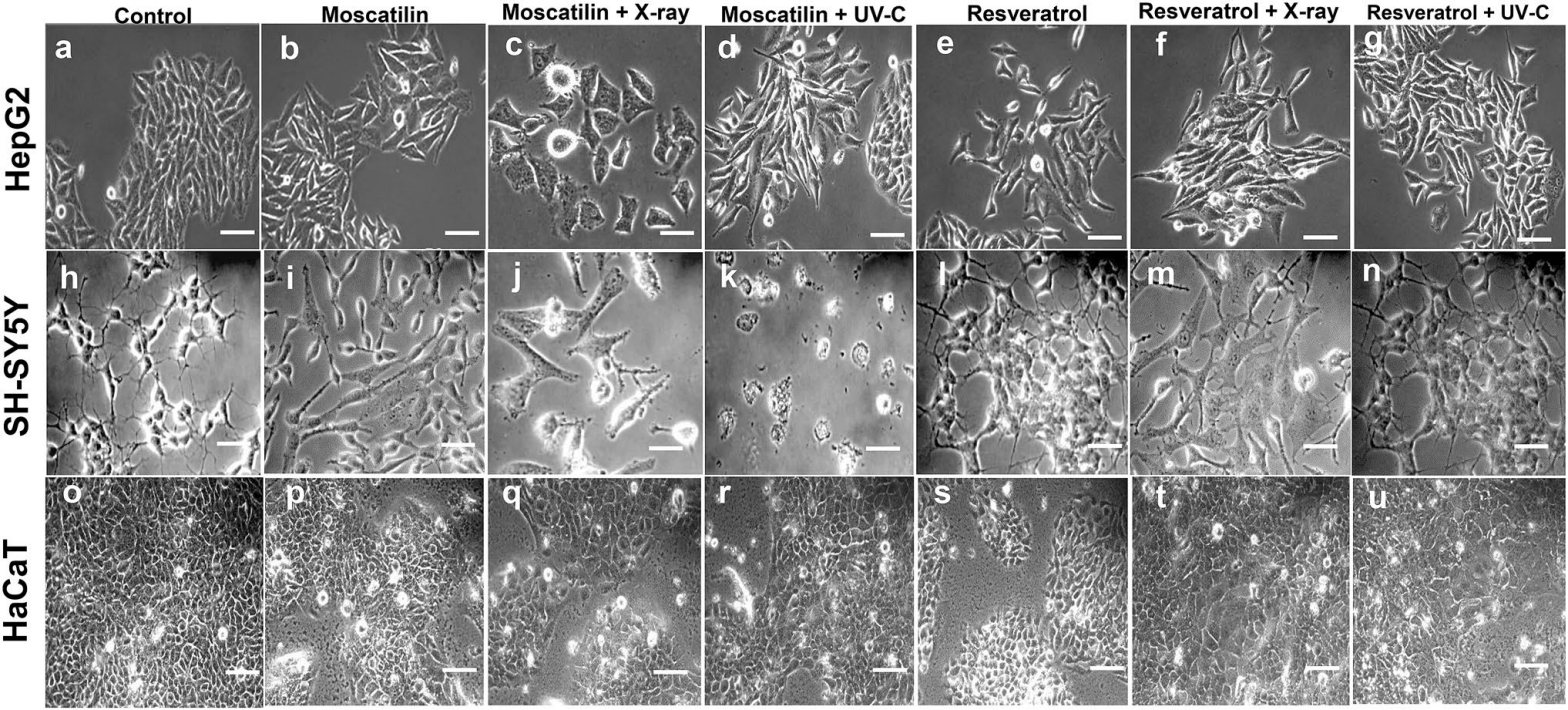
Morphological features of the three studied cell lines (HepG2, SH-SY5Y, and HaCaT) after treatments with Moscatilin and Resveratrol (alone and in combination with minimum effective dose of X-ray and UV-C). a–g depicts HepG2 cells after 72 h of treatments. h–n depicts SH-SY5Y cells after 72 h of treatments. o–u depicts HaCaT cells after 72 h of treatments. The selected X-ray dose was 1 Gy, and that of UV-C was 200 J/m^2^. Rows indicate the cell line type, and the columns indicate different treatments. Scale bar = 100 µm
